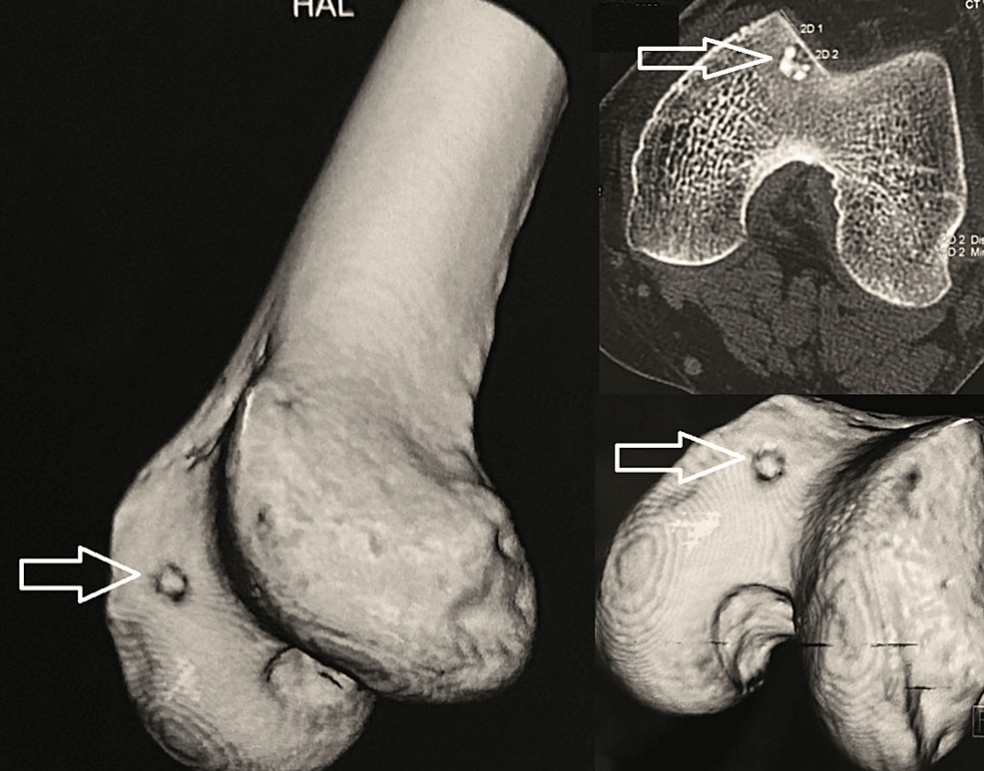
CT scan showing left knee joint Arrows showing the lesion
